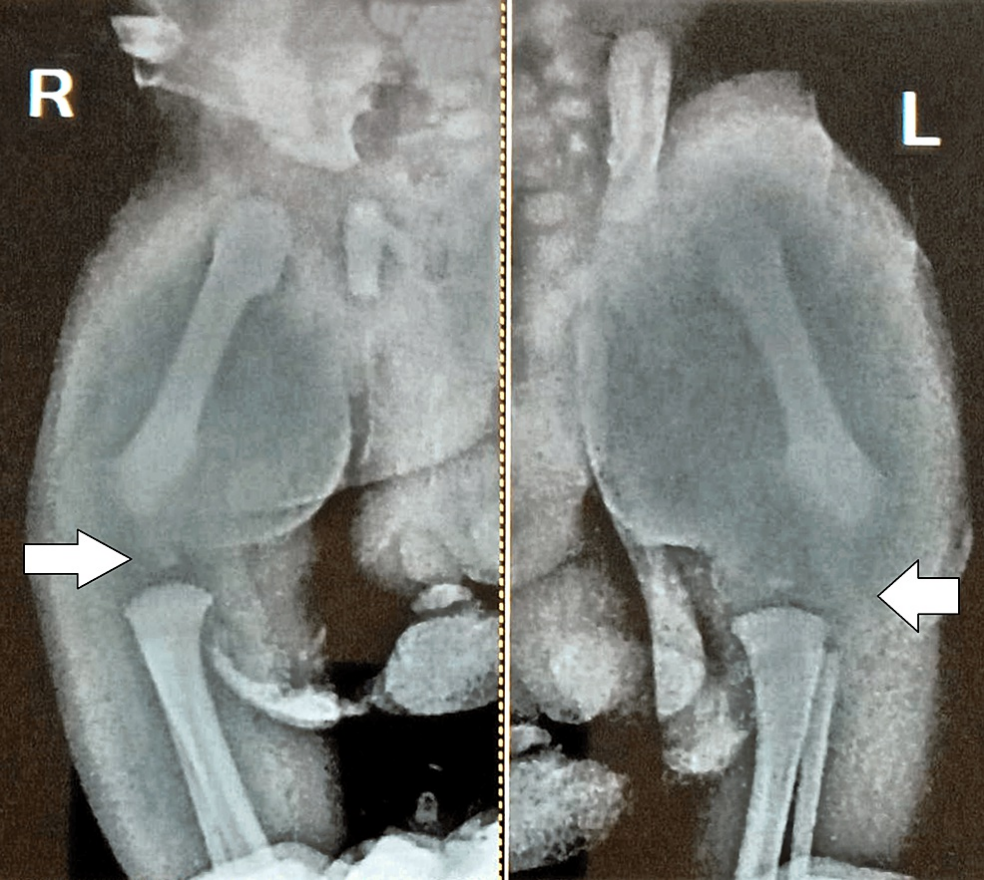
The X-ray of the pelvis revealed left hip joint subluxation (arrows)
